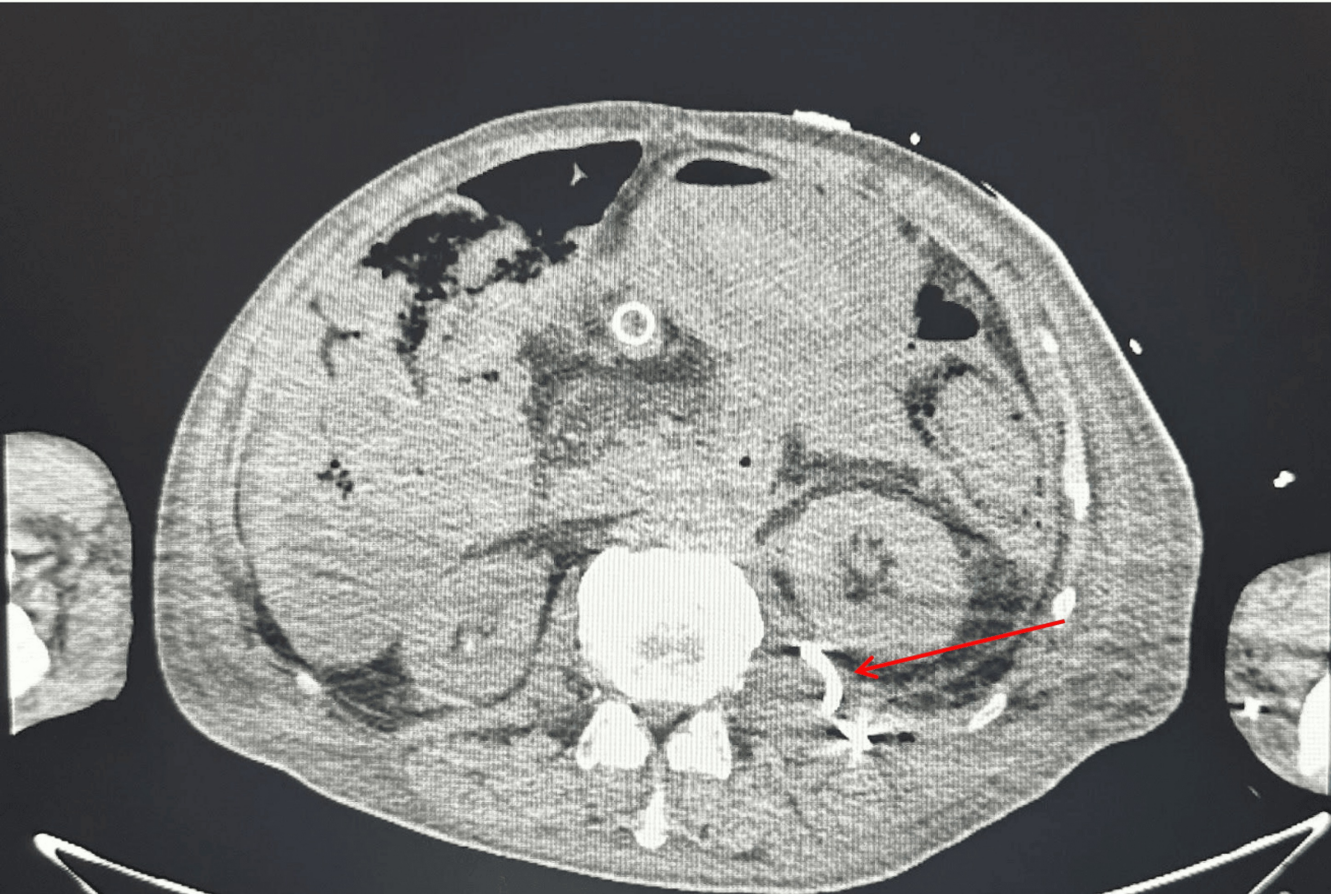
CT of the abdomen and pelvis demonstrating a poorly defined 6 × 3 cm fluid collection with air on the left psoas muscle consistent with psoas abscess following IR drain placement (red arrow) CT: computed tomography, IR: interventional radiology
